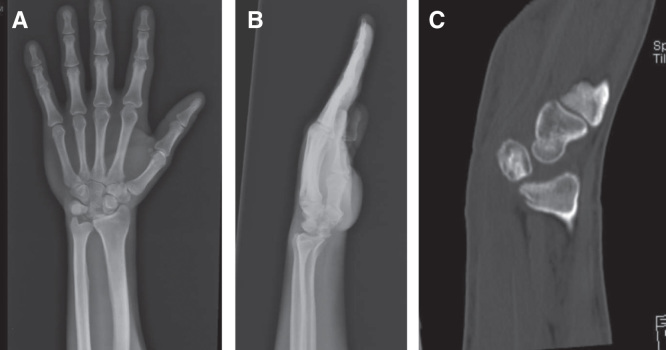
Preoperative X-rays (*a* and *b*) and CT scan (*c*) showing volar dislocation of the lunate bone.
